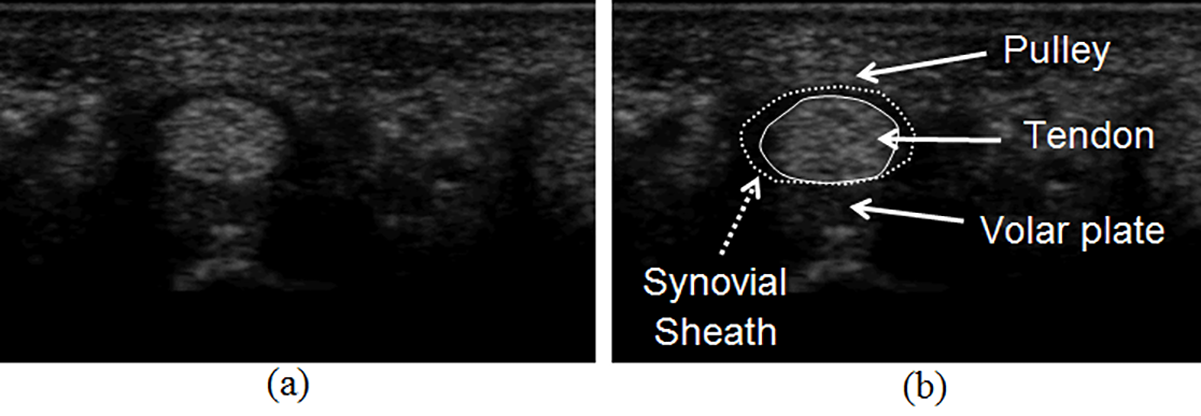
Tendon and synovial sheath in the finger ultrasound image. (a) acquired original image; (b) tendon (solid line), synovial sheath (broken line) area and surrounding tissues.
